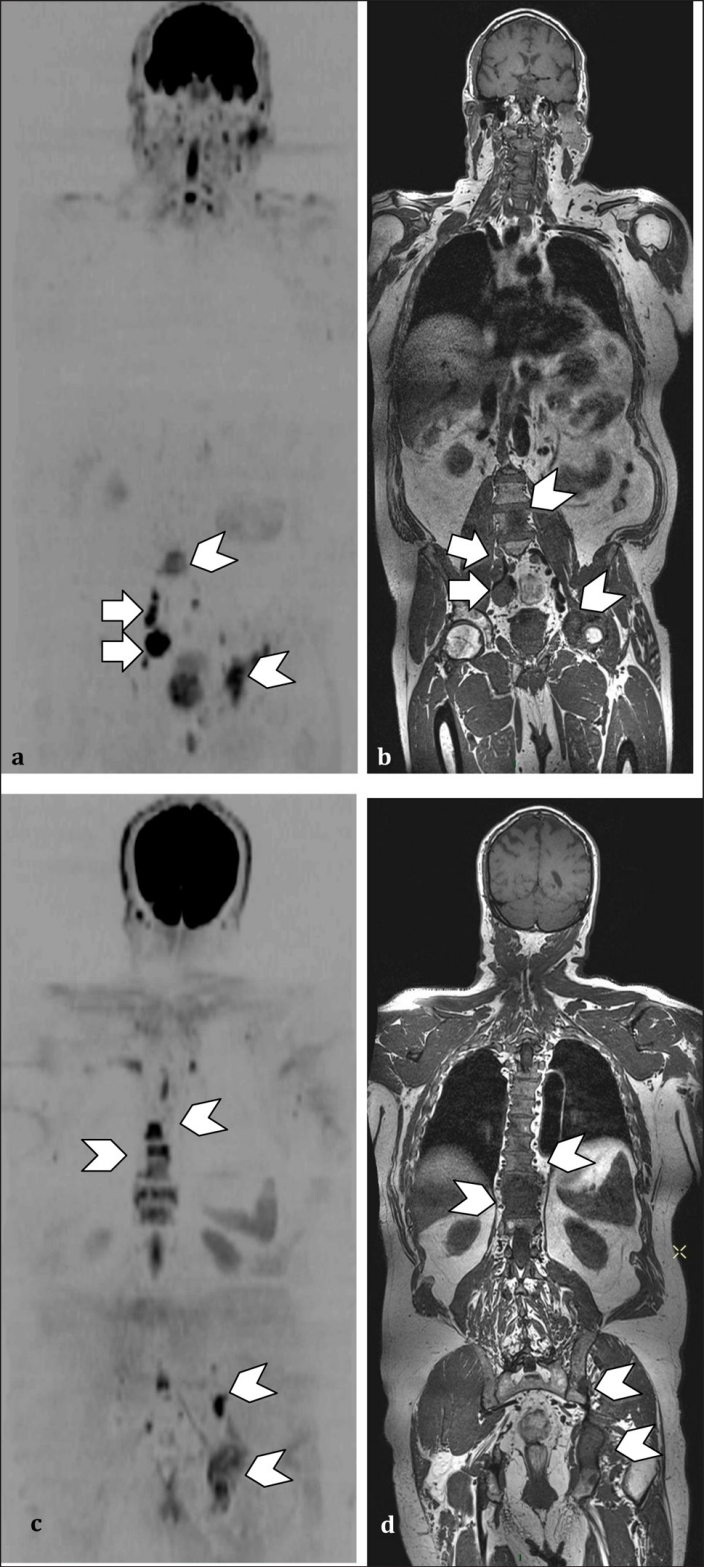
(a and c) Whole body DWI (b and c) Anatomic T1 sequence. PCa patient with nodes (arrows) and bone metastasis (arrowheads) detected by DWI and confirmed by the anatomic sequences.
